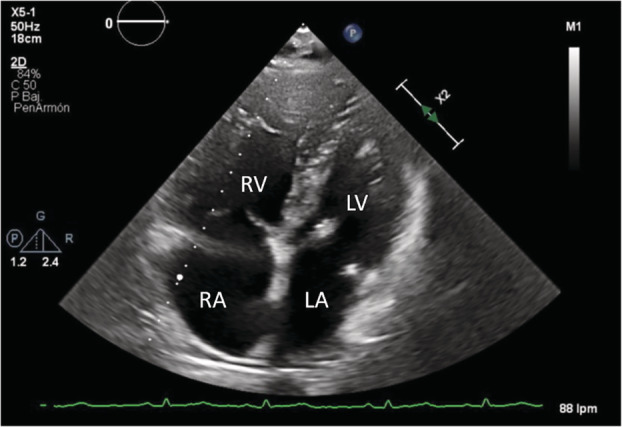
Transthoracic echocardiogram. There is marked dilatation of the right chambers, with displacement of the interventricular septum to the left. (RA, right atrium; RV, right ventricle; LA, left atrium; LV, left ventricle)
